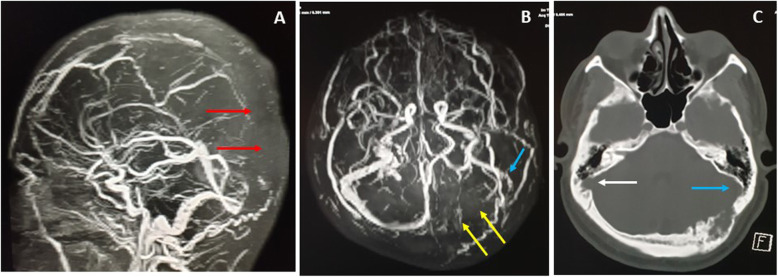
CE-MRV MIP (a) sagittal and (b) axial showed absent enhancement of the superior sagittal dural sinus posteriorly (Red arrow), and the left transverse dural sinus (Yellow arrows). The left sigmoid dural sinus is small/ hypoplastic (Blue arrow). Panel c, is a CT scan bone window showing a shallow left sigmoid groove (Blue arrow) as compared to the right deep groove (White arrow)
